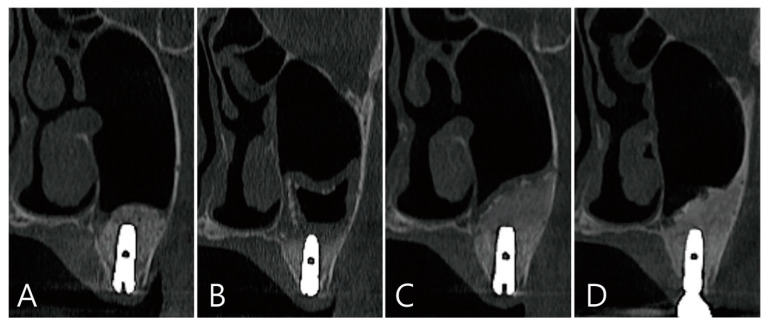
The coronal image of the CBCT scanned at the #27 implant site was examined: (A) in the image taken immediately after surgery, there was no leakage of bone graft particles; (B) however, a very large grafting void appeared in the image taken one week after surgery; (C) this grafting void showed a cystic appearance in the implant apex, although its size was reduced on CBCT taken after 6 months; (D) in CBCT taken 2 years after the grafting void was removed, the grafting void was replaced with new bone.
